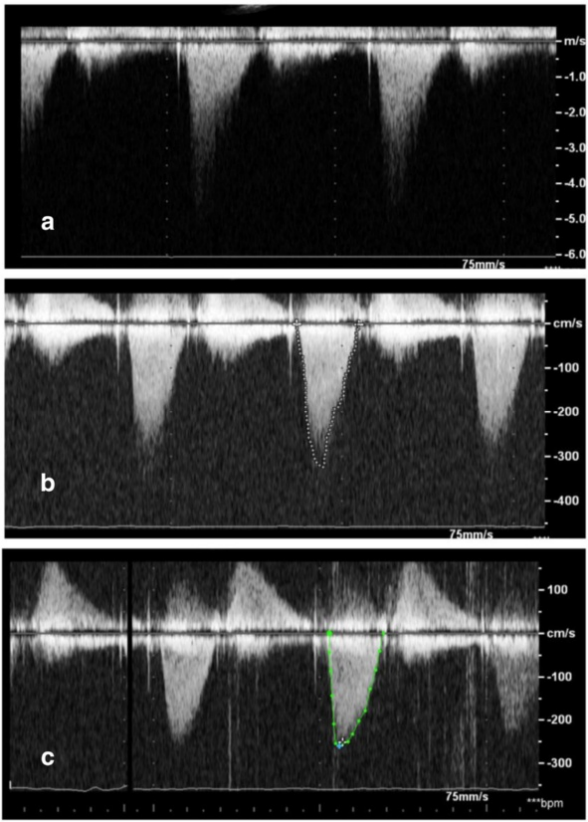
Intra-procedural Trans-esophageal Echocardiography. a Pre-procedure continuous wave Doppler velocity tracing showing elevated gradient across LVOT which is characteristic of dynamic outflow obstruction. b After alcohol septal ablation, continuous wave Doppler velocity still showed elevation of gradient across LVOT. c Continuous wave Doppler velocity showing reduction in gradient across LVOT after injection of glue
